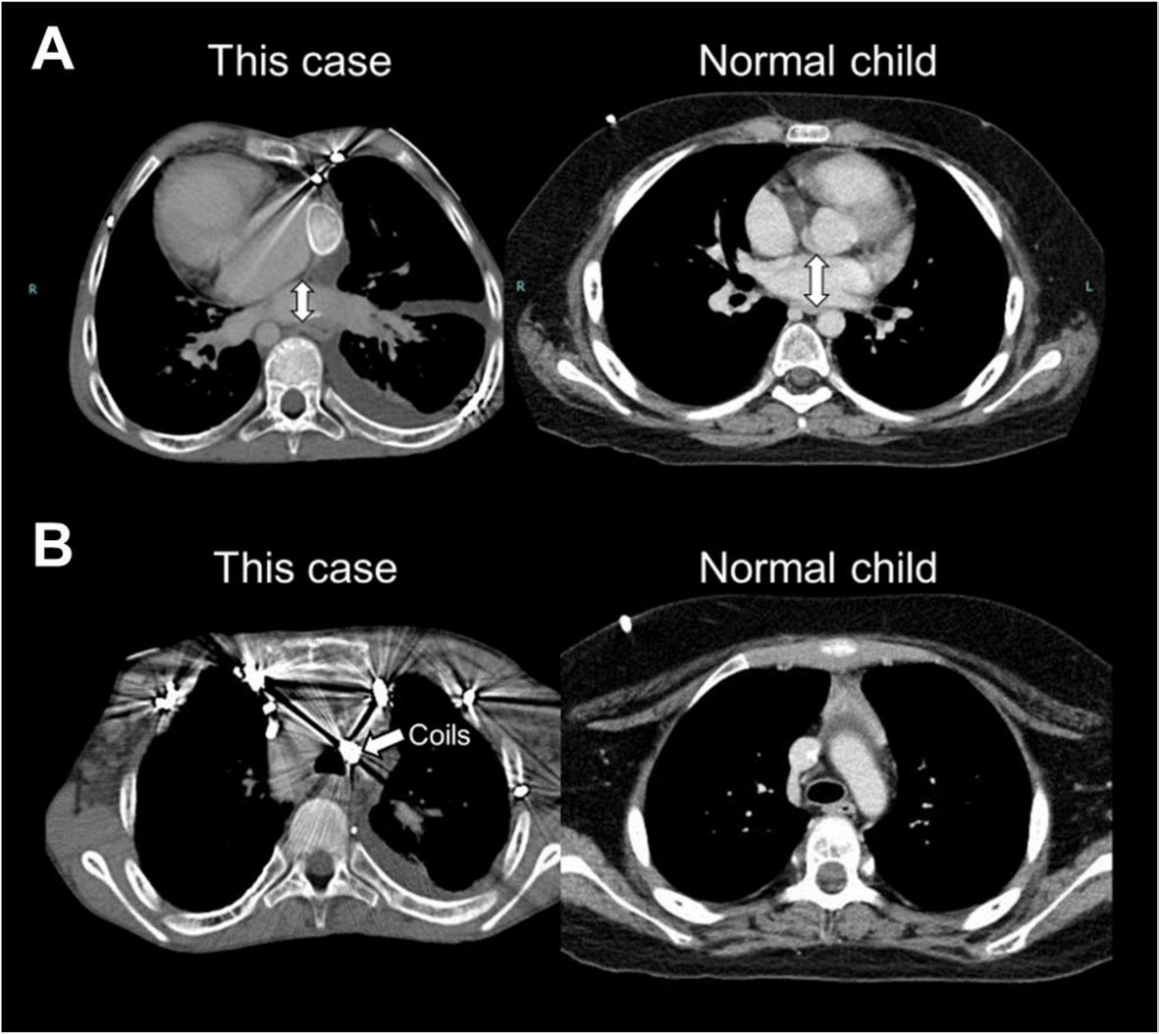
Transverse CT image at the levels of the common pulmonary venous cavity (A) and tracheal bifurcation (B) in this patient (left) and in a normal child (right). Note the smaller anterior-posterior diameter of the common pulmonary venous cavity in this case (left) compared with that of the left atrium in a normal child (right) (A) and the coils for embolizing the major aortopulmonary collateral arteries in front of the tracheal bifurcation (left) (B)
